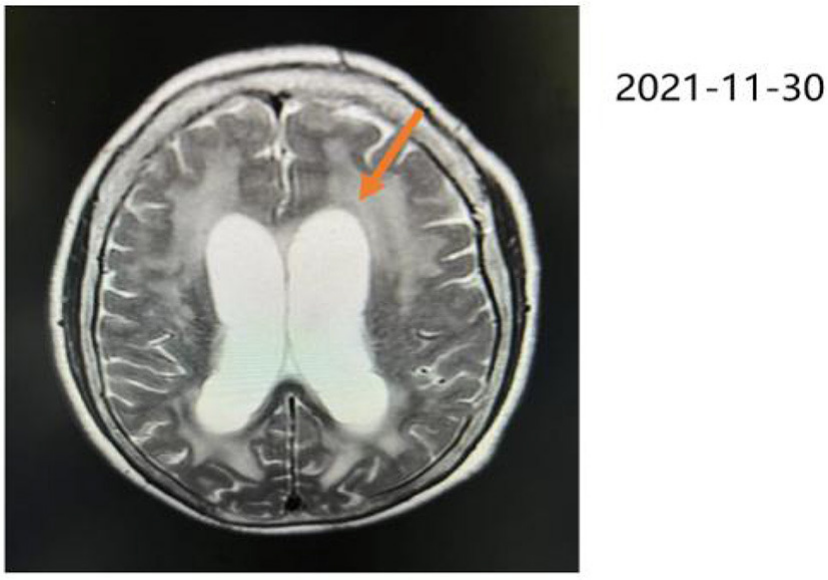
Brain MRI in T2-weighted sequence, taken on 30 November 2021, revealing hydrocephalus, ischemic changes in the bilateral frontoparietal occipital lobe, age-related brain changes, and ventriculomegaly where indicated by the orange arrow.
